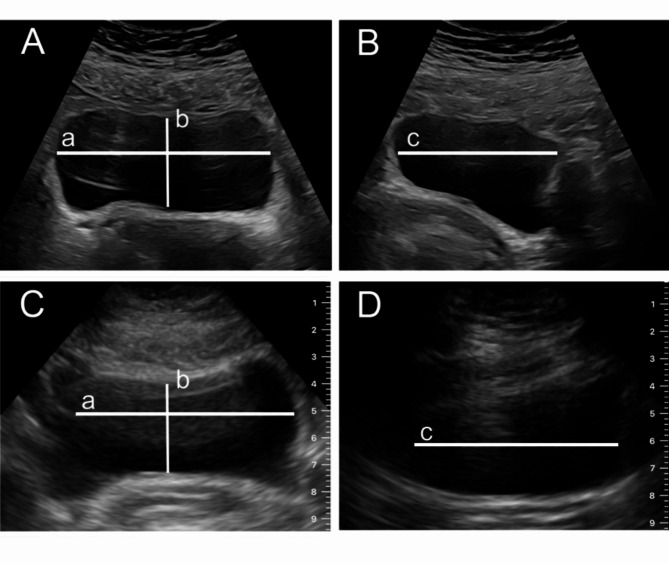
Images of transabdominal ultrasound scans using a standard ultrasound device based on piezo technology (A, B) and a portable ultrasound device based on silicon chips (C, D): Demonstration of the transversal scan plan (A, C) showing the horizontal axis (a) and the sagittal axis (b) and of a sagittal scan plan (B, D) showing the longitudinal axis (c).
